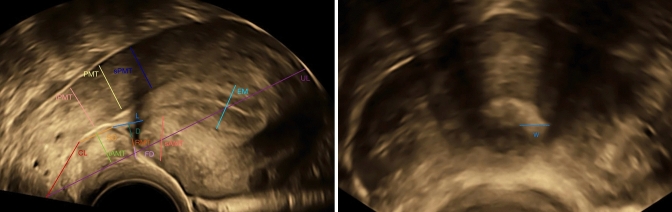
Transvaginal ultrasound of a post-cesarean uterus with the measures of the study: uterine length (UL), cervical length (CL), niche length (L), niche depth (D), niche width (W), RMT, endometrial thickness (EM), scar to internal os distance (SO), anterior myometrial thickness superior (sAMT) and inferior (iAMT) to the scar and the posterior myometrial thickness opposite the scar (PMT), and superior (sPMT) and inferior to it (iPMT)
